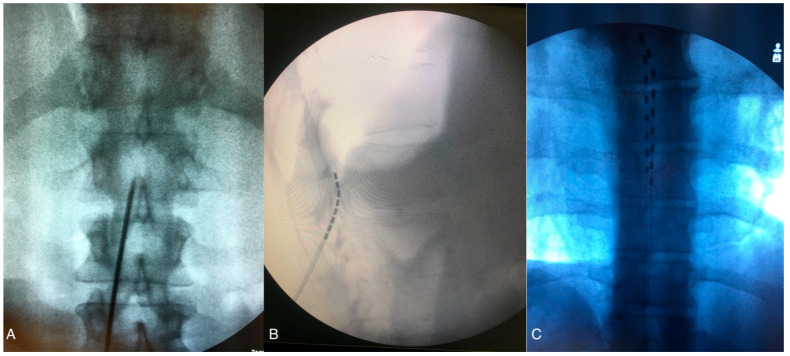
(A) Lumbar spinal X-ray, antero-posterior view: the Tuohy needle is advanced under fluoroscopy through the fascia toward the interlaminar space. (B) Fluoroscopic lateral view of the lumbar spine showing the tip of the needle entering the epidural space and being moved upward. (C) Antero–posterior fluoroscopic image of the two electrodes at T7 level. The figure was created by the authors.
